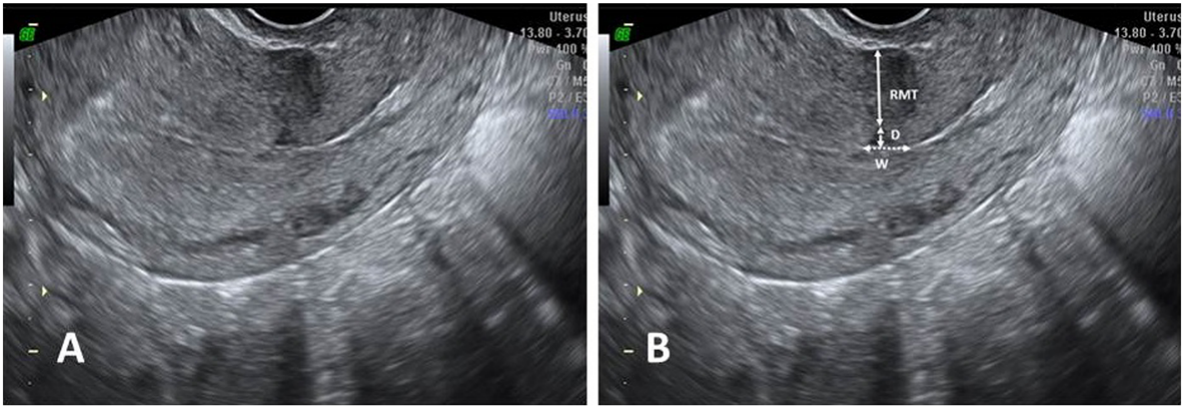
The visualization of the cesarean section scar during transvaginal ultrasound.
